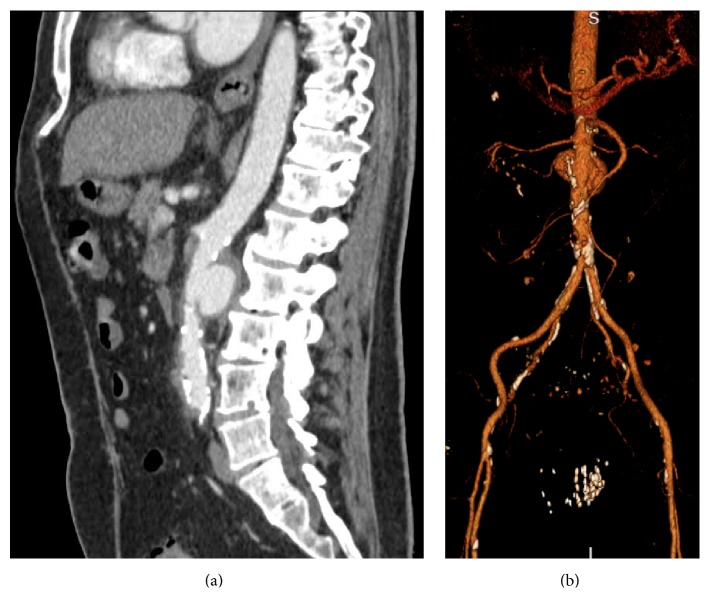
CT image and 3D reconstruction of aneurysm. (a) Sagittal CT shows saccular infrarenal aneurysm with surrounding inflammation. (b) 3D reconstruction demonstrates that the relation of the aneurysm to major abdominal arteries calcifications can be readily identified.
